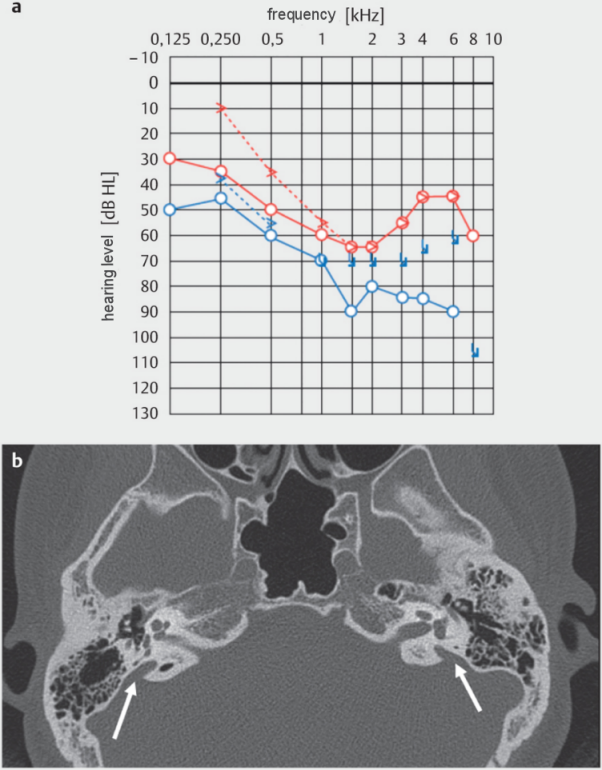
Acute sensory hearing loss after minor trauma in a patient with large vestibular aqueduct syndrome (LVAS; syn.: large endolymphatic duct syndrome or large endolymphatic duct and sac (LEDS); arrows); a: pure tone audiometry (red: right side; blue: left side); b: computed tomography (native, axial); Department of Radiology, University Medicine Halle, courtesy of Prof. S. Kösling.
